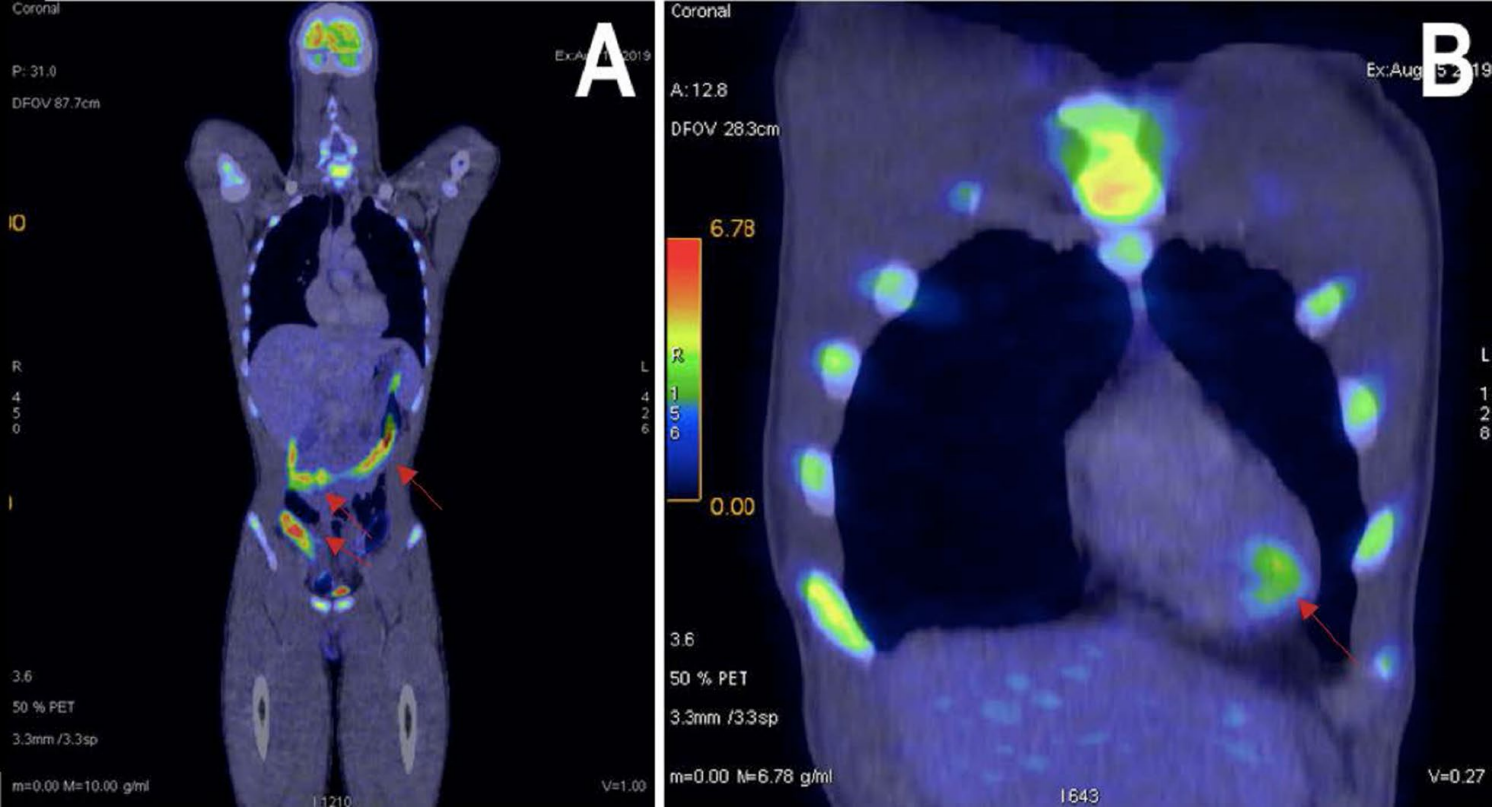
Whole body ^18^FDG PET/CT scan. A Diffuse moderate-intensity of FDG uptake by small and large bowels. B Mild hypermetabolic focus at apical aspect of interventricular septum
